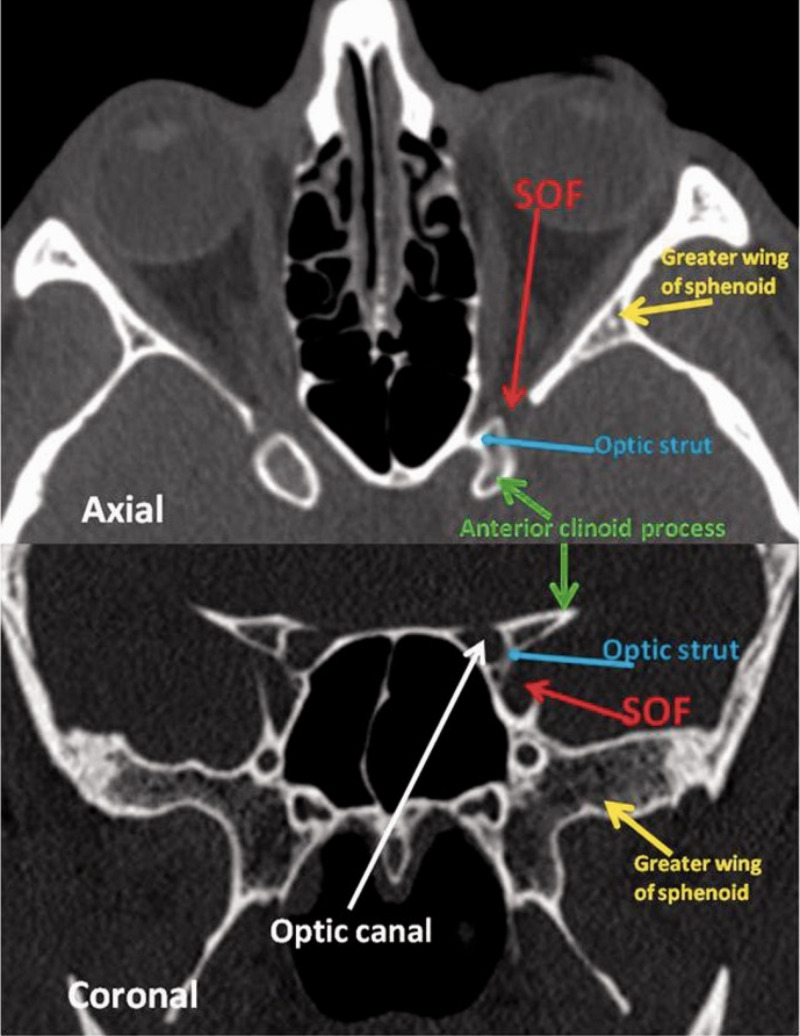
Orbital apex anatomy The axial and coronal CT slices demonstrate the useful clinical anatomy of the orbital apex. Red arrow: SOF; yellow arrow: Greater wing of sphenoid; blue arrow: optic strut; green arrow: anterior clinoid process; white arrow: optic canal; SOF: superior orbital fissure. This image was obtained with consent from The Neuroradiology Journal [3].
